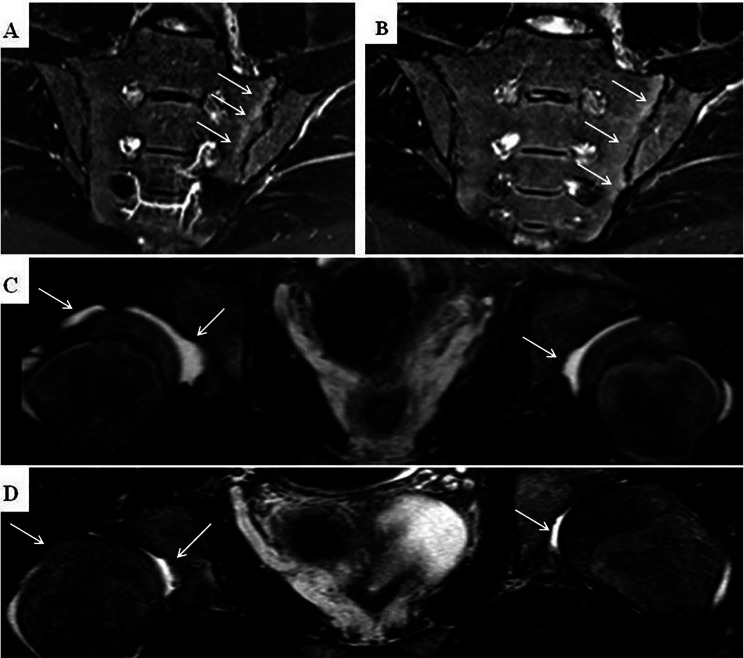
MRI of hips and sacroiliac joints of FOP patients during the study. A – active sacroiliitis in 18 years old male patient before tofacitinib therapy (the subchondral bone marrow edema noted by the arrows). B – the same patient after 1 year of tofacitinib treatment (reduction of the subchondral bone marrow edema, arrows). C – MRI evidence of bilateral hips effusion in 12 years old male patient before tofacitinib therapy (arrows). D – the same patient 1.5 years after tofacitinib treatment, regression of synovial effusion in the hip joints (arrows)
